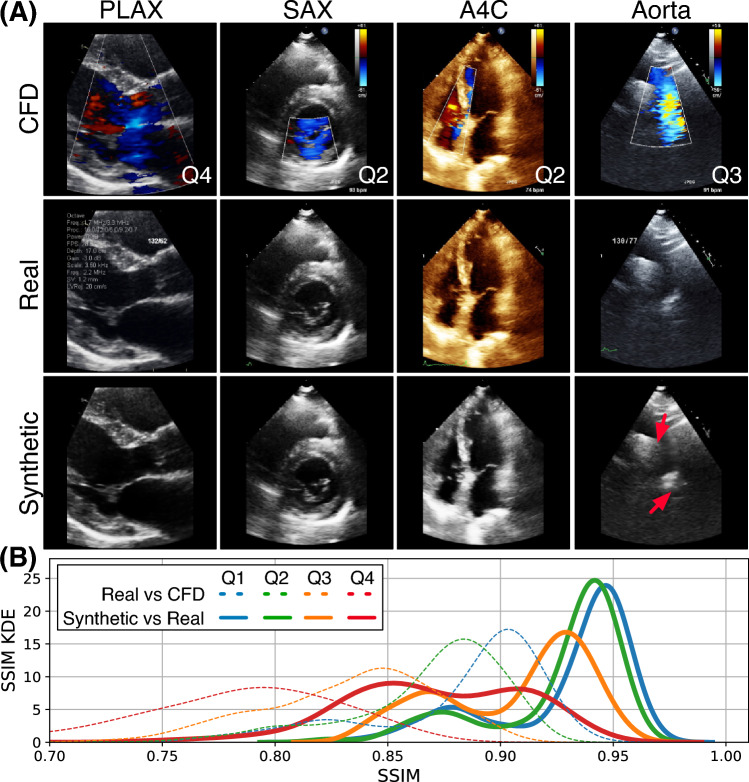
GAN pipeline generates realistic synthetic B-mode videos with high structural similarity for adult cardiac ultrasound. (A) Examples showing CFD, corresponding ground-truth B-mode, and synthetic B-mode. Anatomic structures underneath the color signal are reconstructed (red arrows) across quartiles of CFD coverage (indicated in lower right-hand corner of each CFD example). (B) SSIM kernel density estimates (KDE) comparing ground-truth B-mode to CFD (dashed line) and to synthetic B-mode (solid line). Q1 represents videos with the lowest CFD coverage (see Methods), and Q4 the highest. *PLAX* parasternal long axis, *SAX* short axis, *A4C* apical 4-chamber, *Aorta* aortic arch view.
